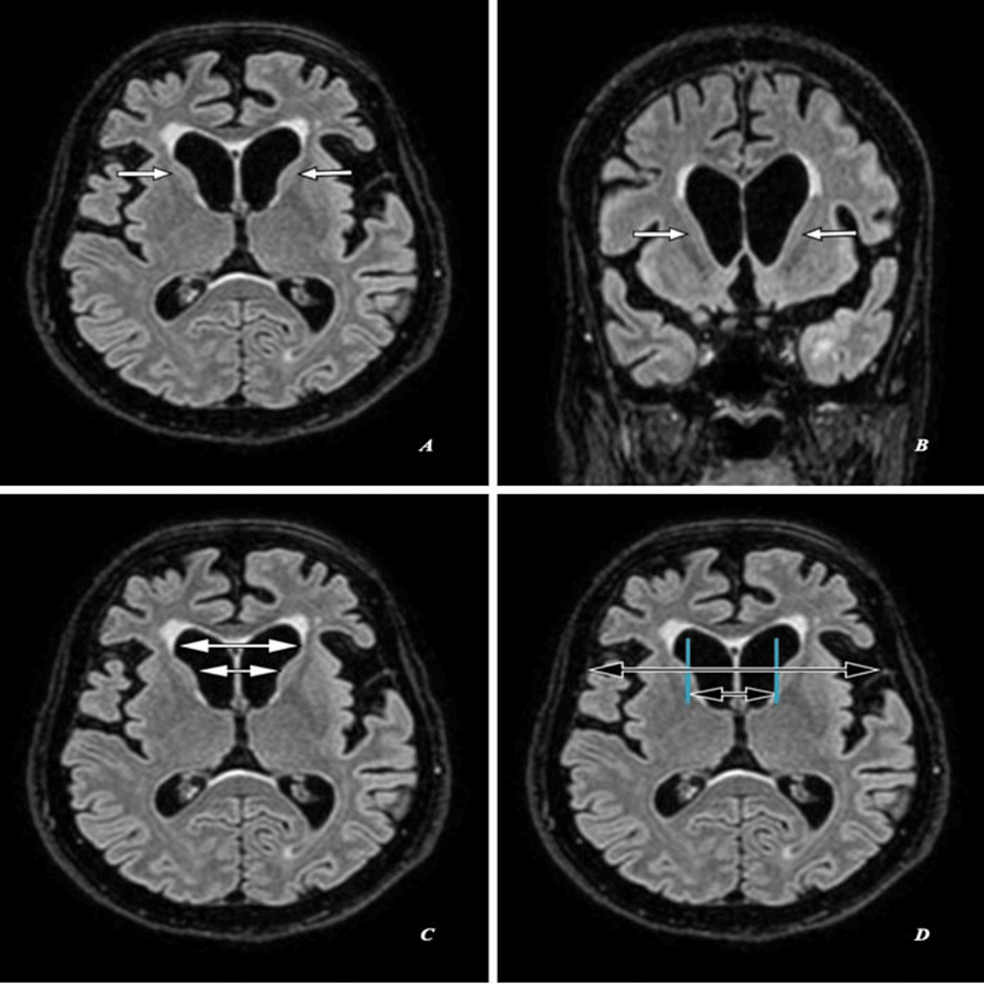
Magnetic resonance imaging (MRI) brain (fluid attenuation inversion recovery/FLAIR): (A,C,D) Axial sections and (B) coronal section of the same patient. (A,B) Shows head of bilateral caudate nuclei mild atrophy with ex-vacuo mild dilatation of frontal horns of lateral ventricles (short white arrows). (C) Shows frontal horn width (large double white arrow) to intercaudate distance (small double white arrow) ratio (FH/CC) of 1.6, mildly decreased. (D) Shows an intercaudate distance (small double black arrow) to inner table width (large double black arrow) ratio (CC/IT) of 0.24, mildly increased.
